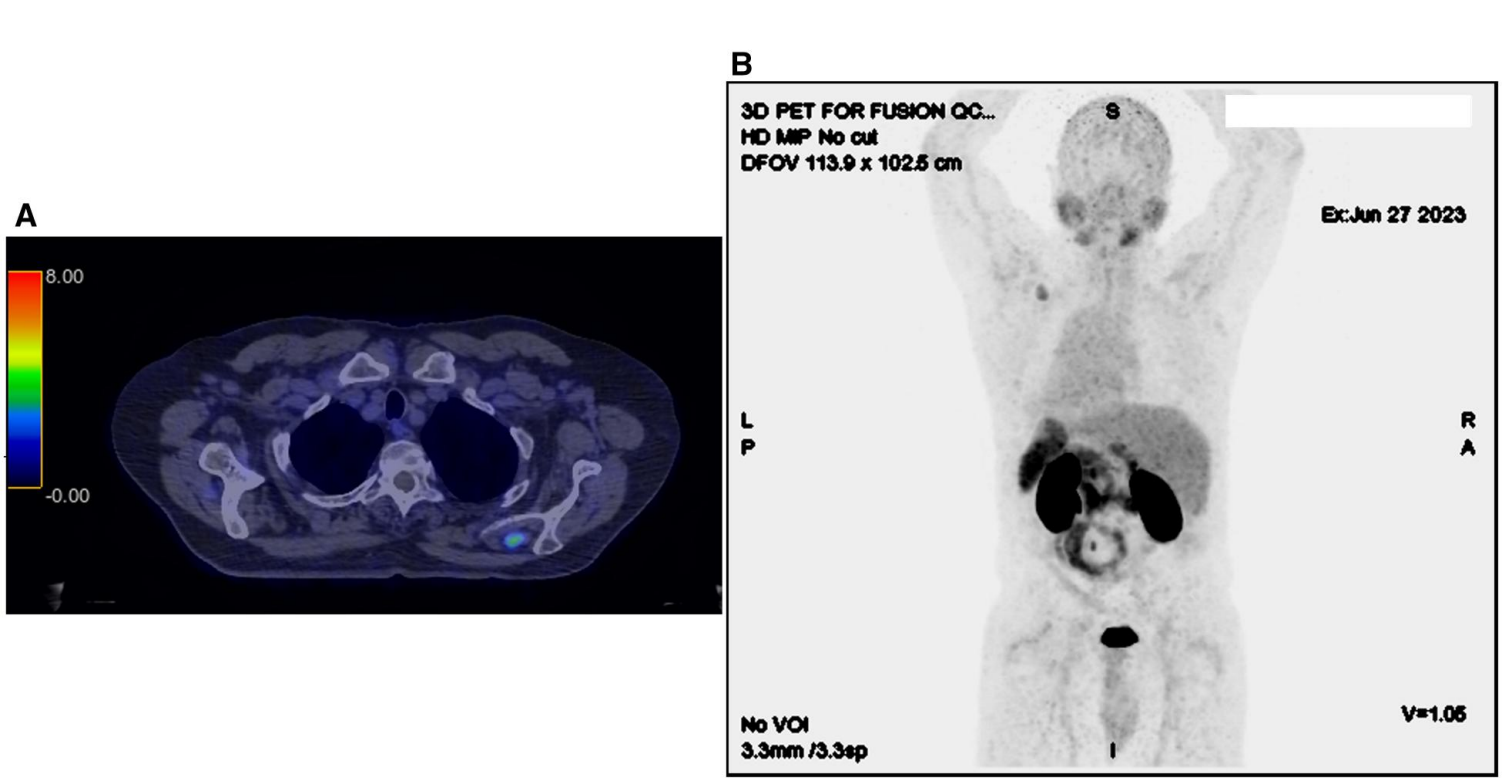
Whole body PSMA-PET/CT. (A) Moderate PSMA-PET avidity in the left supraspinatus. (B) No other PSMA-avid lesions.
